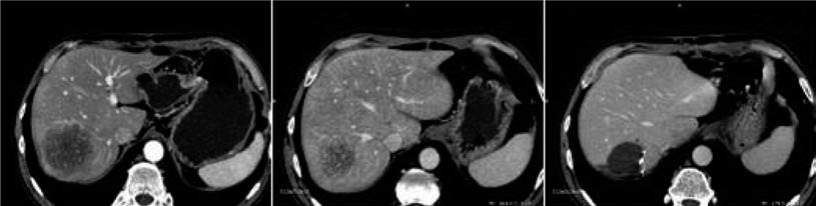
(a) CT before combined systemic and intra-arterial chemotherapy—large lesion the right hepatic lobe, more than 7 cm in diameter; (b) CT after two cycles of combined chemotherapy—the lesion is smaller, with a diameter of less than 4.5 cm; (c) CT after right hepatic lobe resection: post-surgical small bile collection (arrow) and the evidence of left hepatic lobe hypertrophy
